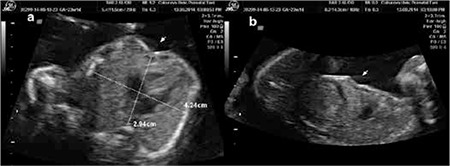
Ultrasonographic images of pectus excavatum, a) Axial thoracic section, pectus severity index is calculated by the ratio of the lateral diameter of the chest to the distance between the sternum and spine at the point of maximal depression (pectus severity index: 4.24 cm/2.94 cm=1.44), b) Sagittal thoracic section (arrows indicate the defect)
